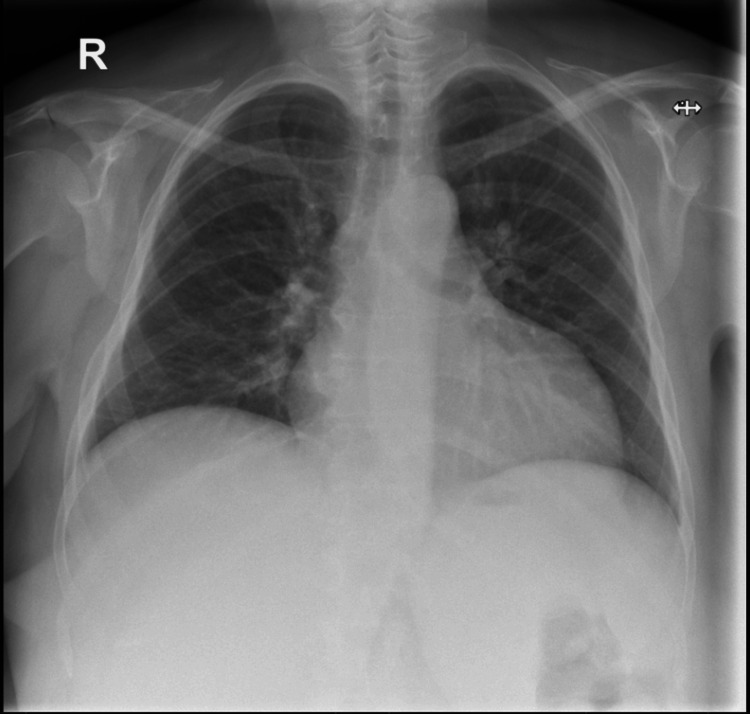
Chest X-ray done on day 1 shows a clear lung field and cardiothoracic ratio of 15/28 cm
